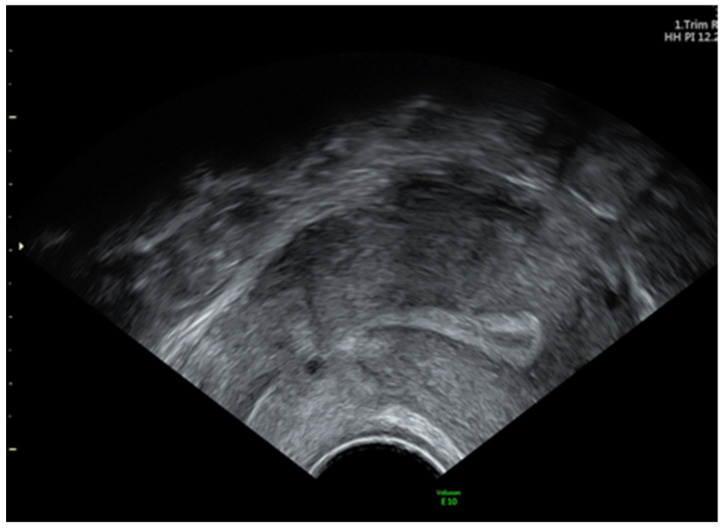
Transvaginal ultrasound image of the uterus in the sagittal plane—6 months after the surgery, demonstrating a completely restored cesarean scar site.
